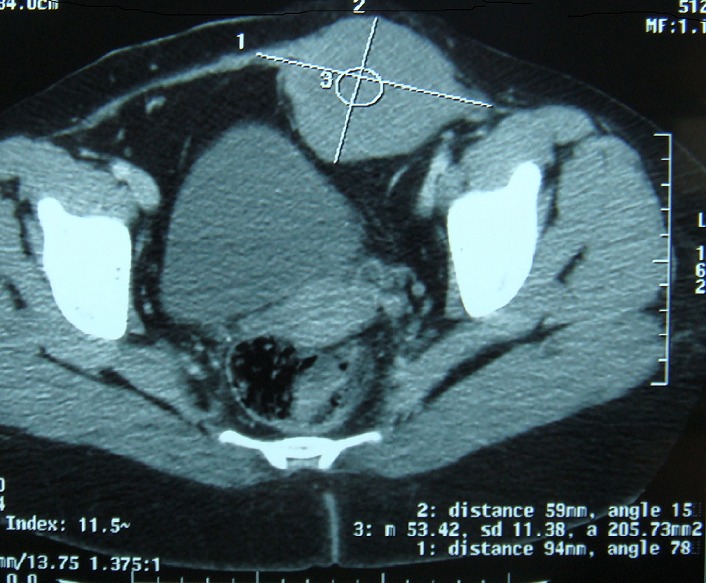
Abdominal CT scan shows an heterogeneous mass of the left iliaca fossa
